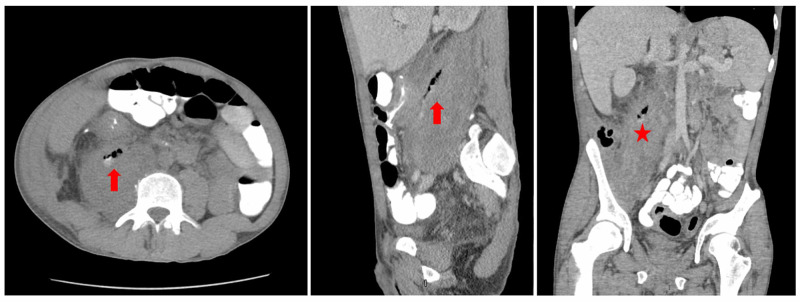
Computed tomography of the abdomen with and without oral and intravenous contrast showing a fistulous connection between the terminal ileum and the right psoas major (star). This was markedly edematous and inflamed and contained phlegmon/abscess (arrow).
